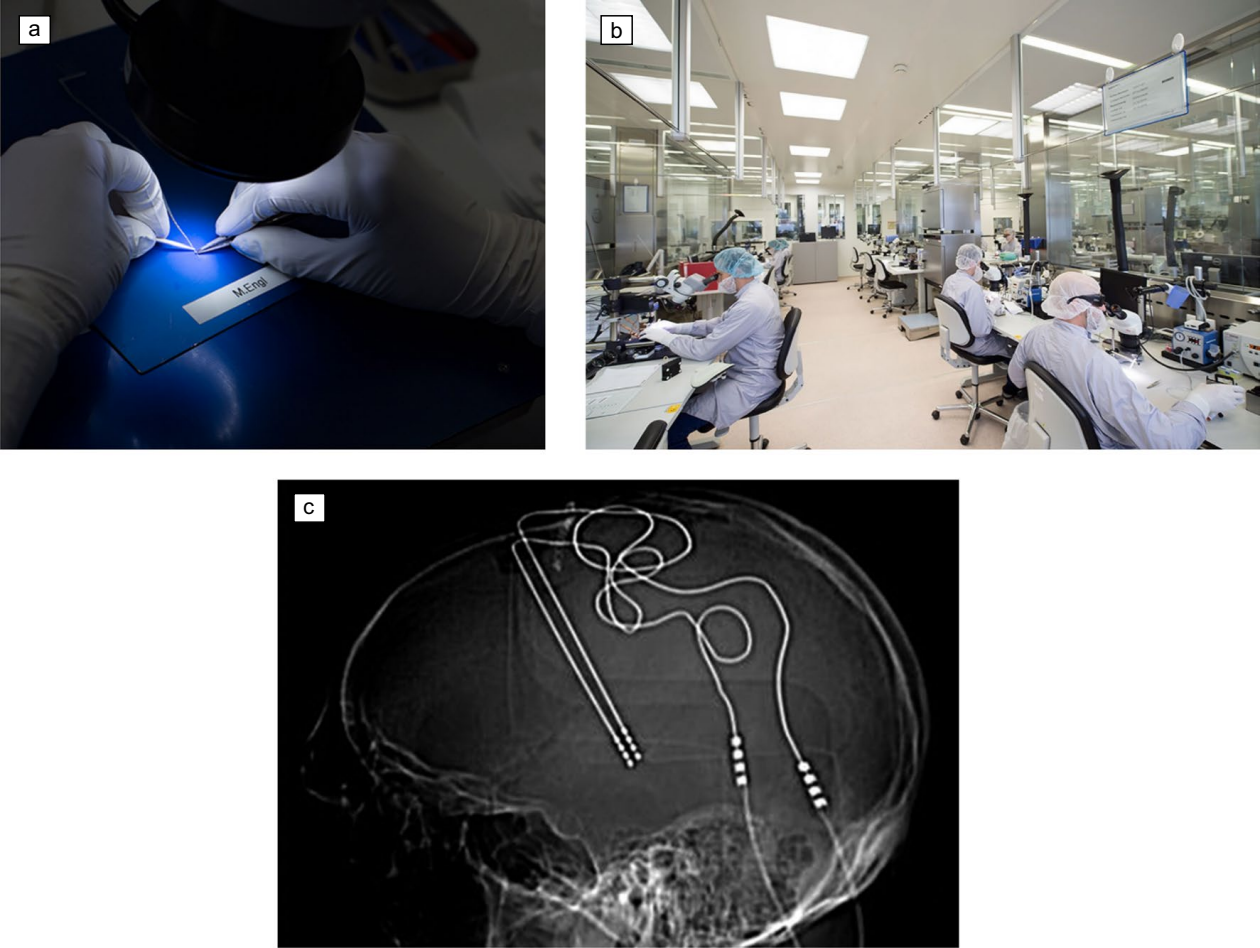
(a, b) Fabrication of current implants for bioelectronic medicine. The process involves a manual assembly line. Reprinted with permission from Reference 19. © 2016 MED-L. (c) Postoperative lateral x-ray shows deep brain stimulation leads implanted in the left and right subcallosal cingulate region. Reprinted with permission from Reference 26. © 2019 *Proceedings of the National Academy of Sciences*.
